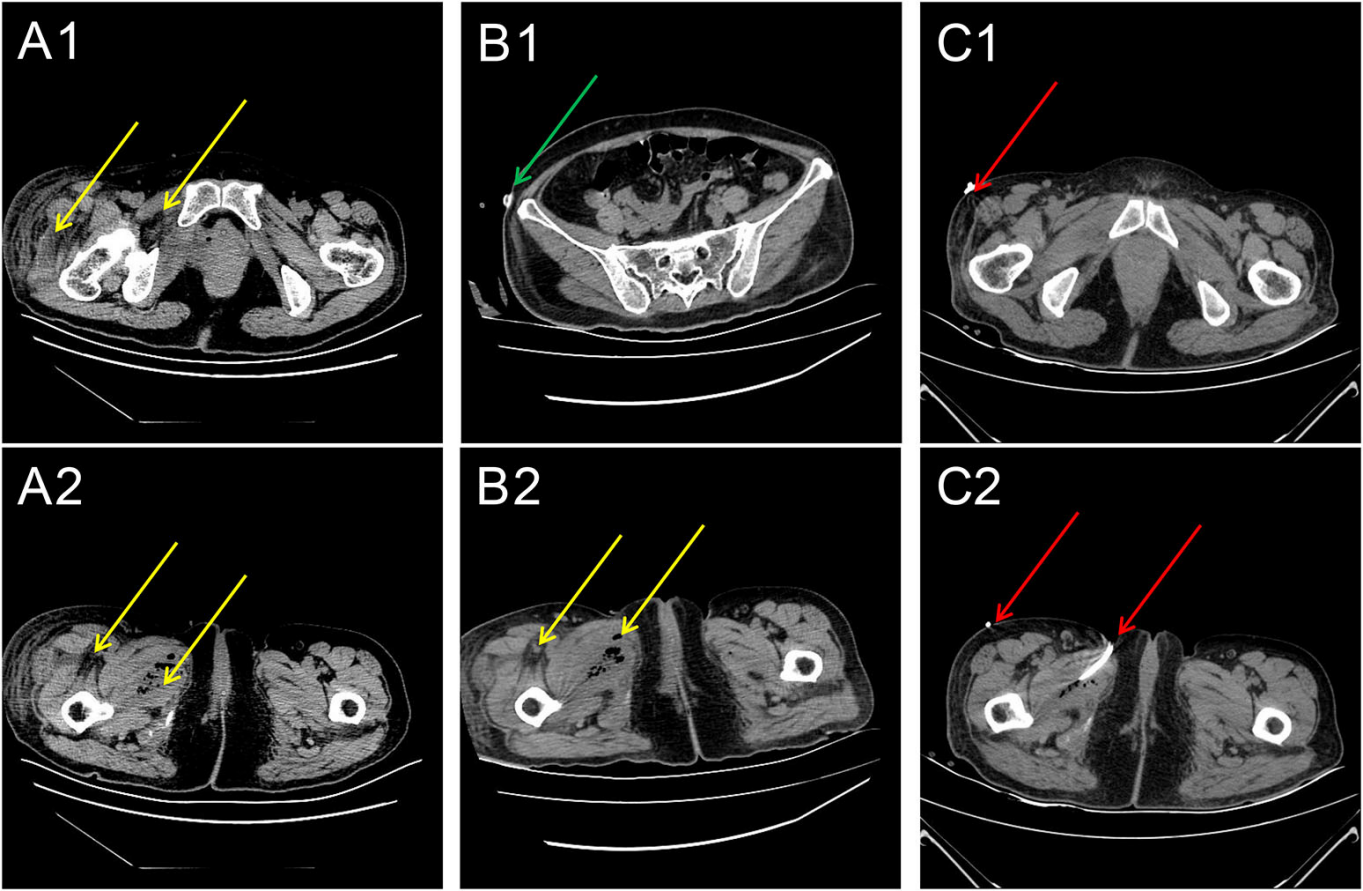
Series of computed tomography (CT) images (A) 1 week, (B) 2 weeks, and (C) 3 weeks after the sling procedure. (A1,A2) The CT scans of the pelvis and thigh regions indicate that the pelvic floor and thigh root muscle cavities have multiple gas shadows (yellow arrows). (B1,B2) The CT scans of the pelvis and thigh for assessing the infection status. The green arrows indicate the location of the drainage tube. The location of the gas (yellow arrow). (C1,C2) The CT scans of the pelvis and thigh for assessing the infection status. The red arrows indicate the location of the corrected drainage tubes. The location of the gas (yellow arrow).
